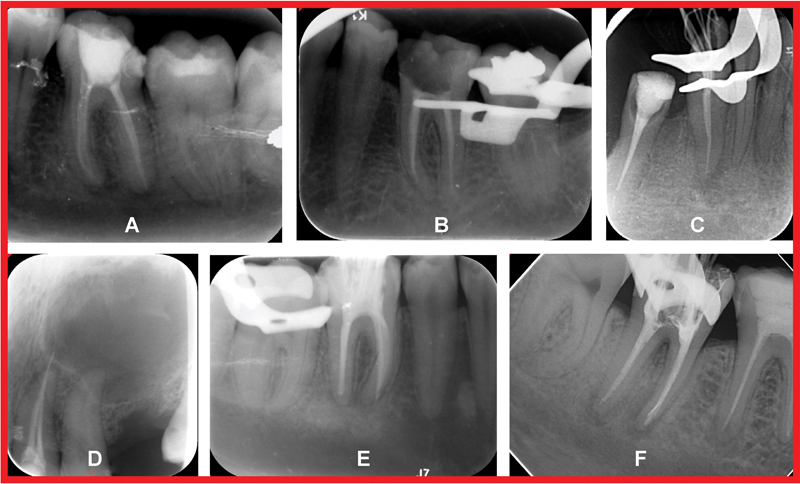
Samples of radiographic images used for the evaluation of the root canal fillings. (
